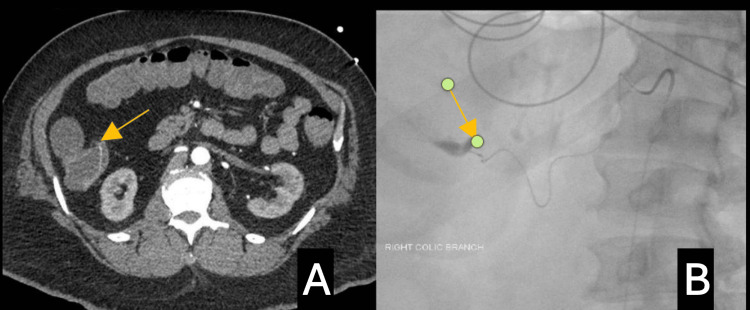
A CT angiogram of the abdomen shows contrast extraversion within the ascending colon (Figure 3A). Interventional radiology (IR)-coil embolization of the right colic artery with a suspected Dieulafoy lesion is seen (Figure 3B).
